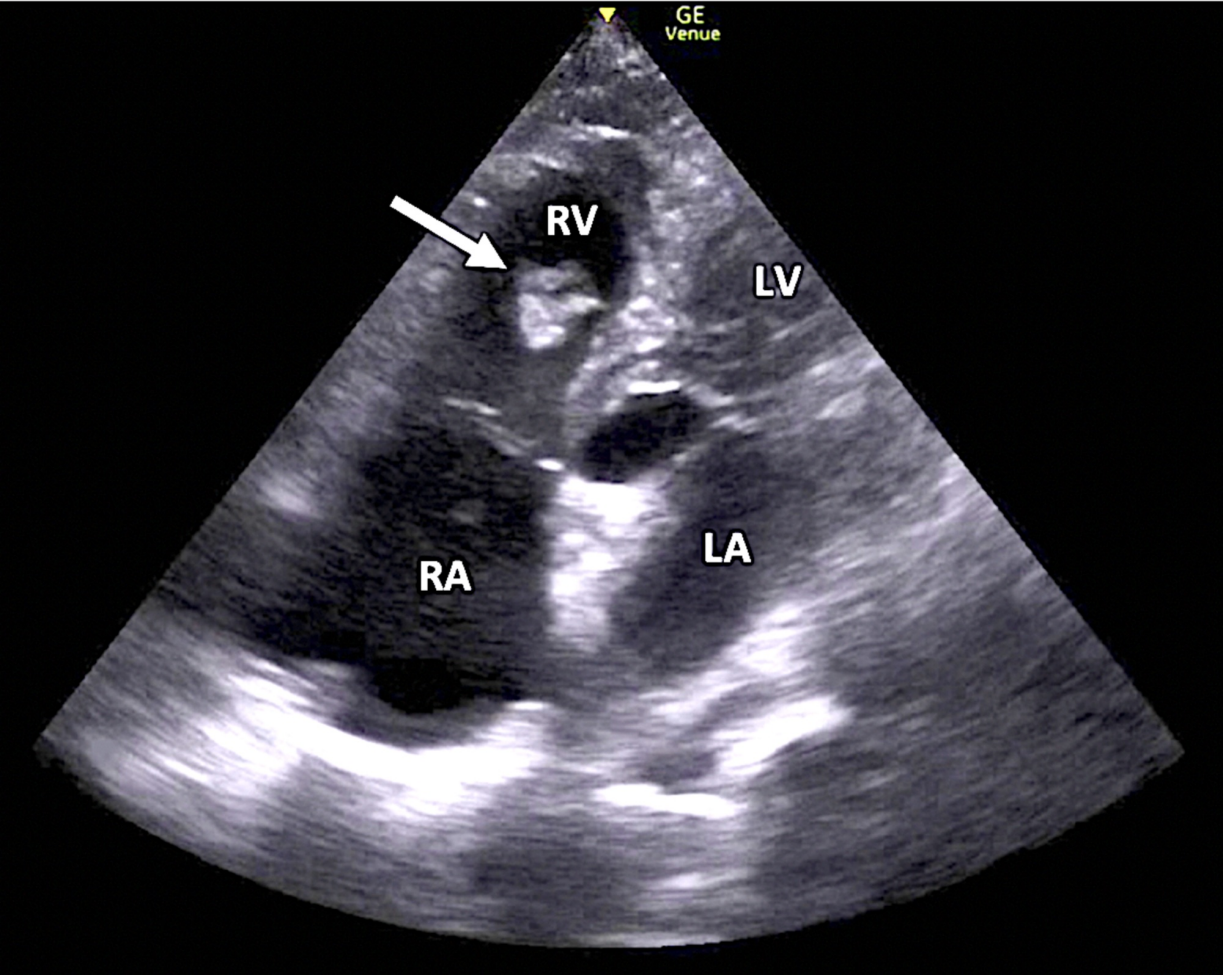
Focused cardiac ultrasound (apical four-chamber window) demonstrating a right heart thrombus (arrow). RV, right ventricle; LV, left ventricle; RA, right atrium; LA, left atrium.
